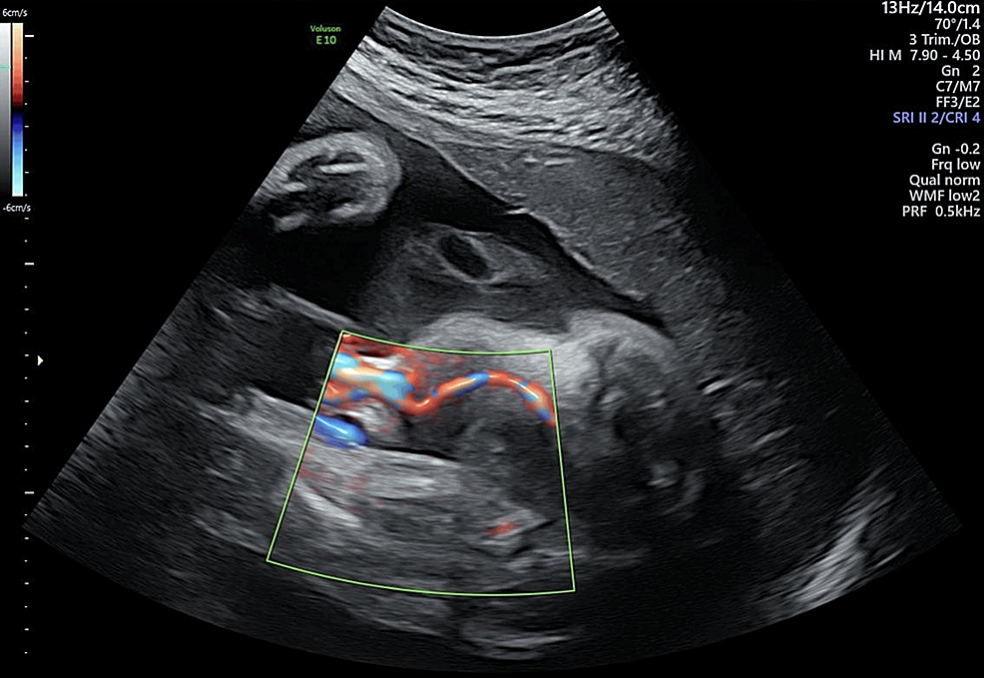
Single umbilical artery at 30+6 weeks (using a color Doppler ultrasound diagnostic system; Voluson™ E10, GE HealthCare, USA)
